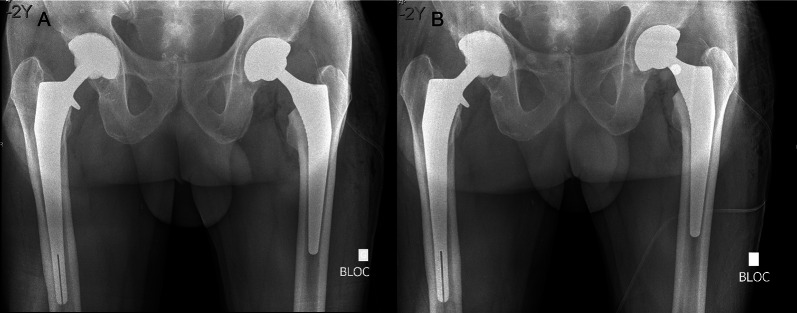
X-ray after postoperative index (A) and revision surgery (B). Change of two sizes (+4 mm to −4 mm) with an 8 mm LLD. *LLD* leg length discrepancy
